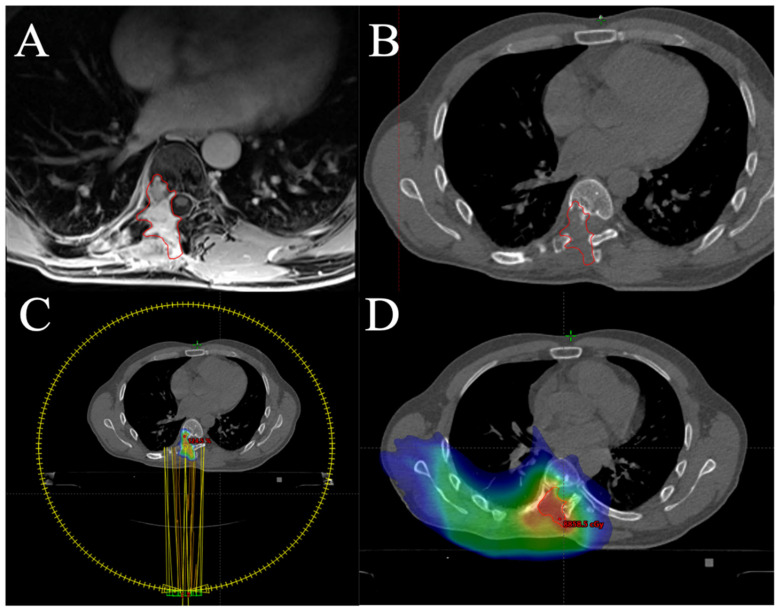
Stereotactic body radiotherapy (SBRT) for in-field recurrent high-grade mesenchymal chondrosarcoma (confirmed in next-generation sequencing sarcoma panel). In 2015, the patient underwent neoadjuvant chemotherapy followed by surgery, adjuvant radiochemotherapy (50.4 Gy in 1.8 Gy fractions with vincristine and dactinomycin combination chemotherapy), and chemotherapy according to EIAO regimen (etoposide, ifosfamide, vincristine, and dactinomycin). In 2019, he experienced local recurrence (LR) in the paraspinal area, which was resected in 2020. Five months later, the second LR was observed (A,B). The patients received SBRT (C) planned on fusion with magnetic resonance imaging and previous treatment plan (D). Volumetric arc therapy was used to deliver 30 Gy in five fractions prescribed on 80% isodose (C). Cord tolerance doses were compliant with the report published by Sahgal et al. [162]. Photos by Mateusz J. Spałek.
